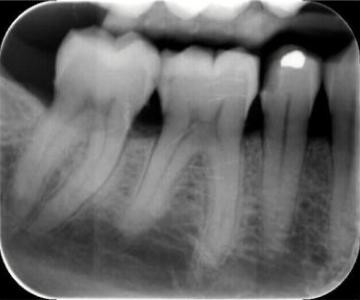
Endo-oral X-ray of the fractured dental amalgam.
